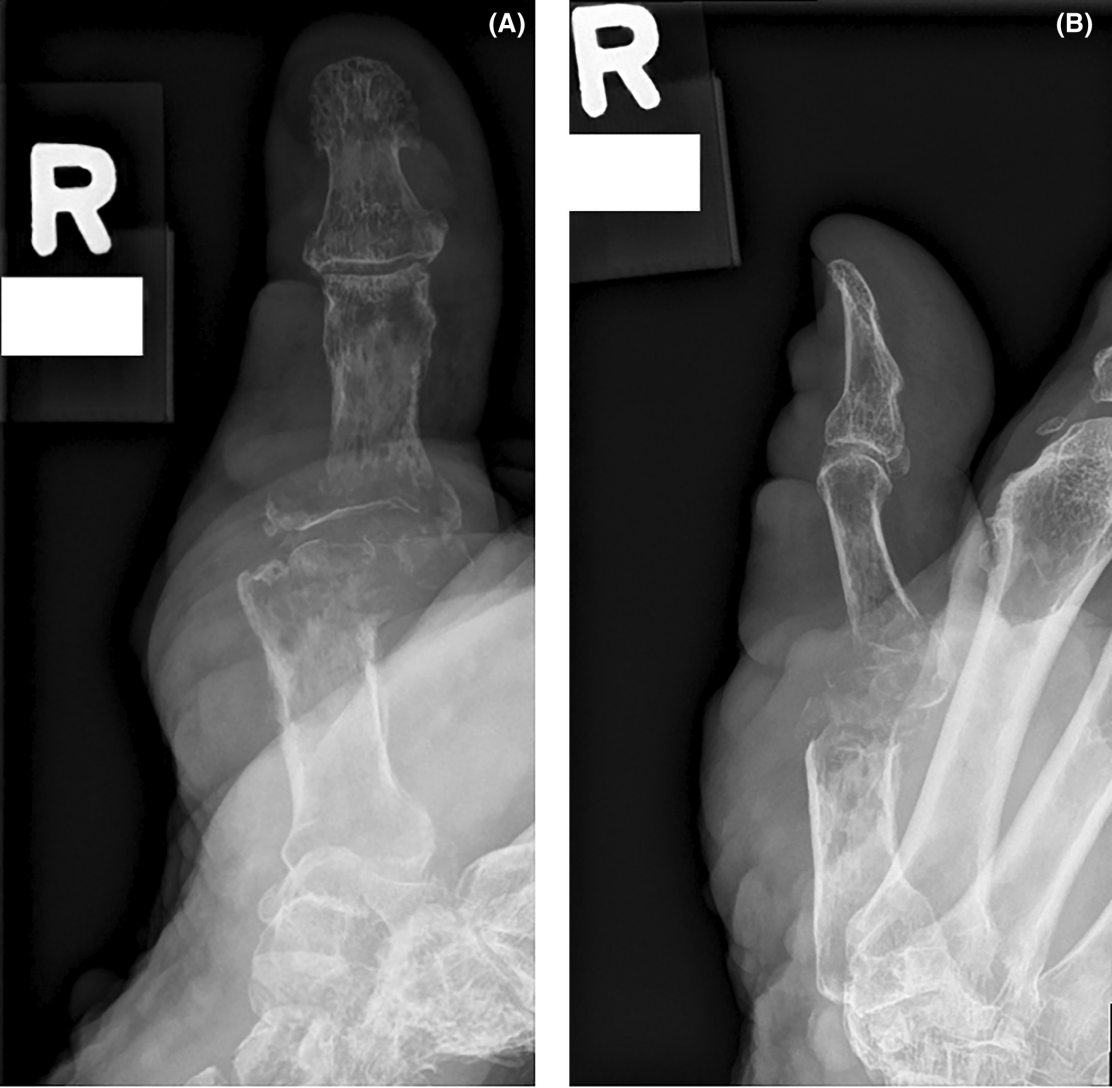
Radiographs right thumb. (A) Posterior–anterior view of right thumb (left image). (B) Lateral view of right thumb (right image).
